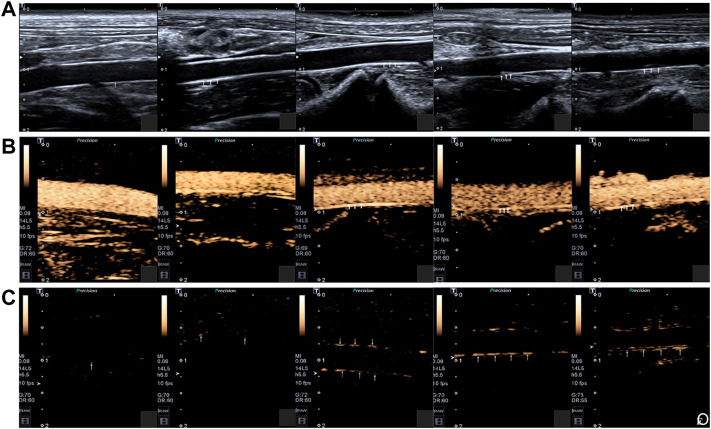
(A) Two-dimensional ultrasonography images of the abdominal aorta of rabbits in different groups. (B) SonoVue ultrasonic contrast images of the abdominal aorta of rabbits in different groups. (C) ICAM-1-targeted nano ultrasonic contrast images of the abdominal aorta of experiment rabbits in different groups. Five rabbits in each group: control group; week-4 group; week-8 group; week-12 group; week-16 group. The intima-media membrane and the plaque on the wall were indicated by the arrows (Copyright permission from Springer Nature) (Li et al., 2021).
